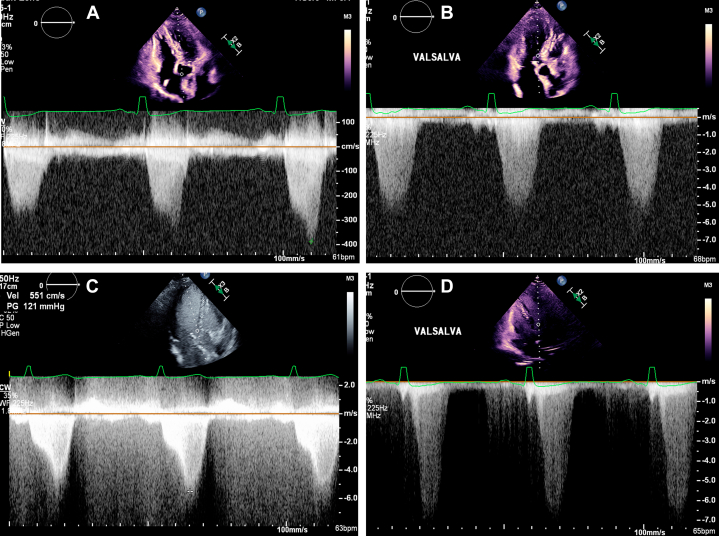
LVOT Gradients at Rest and With Valsalva Maneuver LVOT continuous-wave Doppler (A) at rest and (B) during Valsalva. LVOT contrast-enhanced continuous-wave Doppler (C) at rest and (D) during Valsalva. The maximum provoked gradient was 170 mm Hg. LVOT = left ventricular outflow tract.
